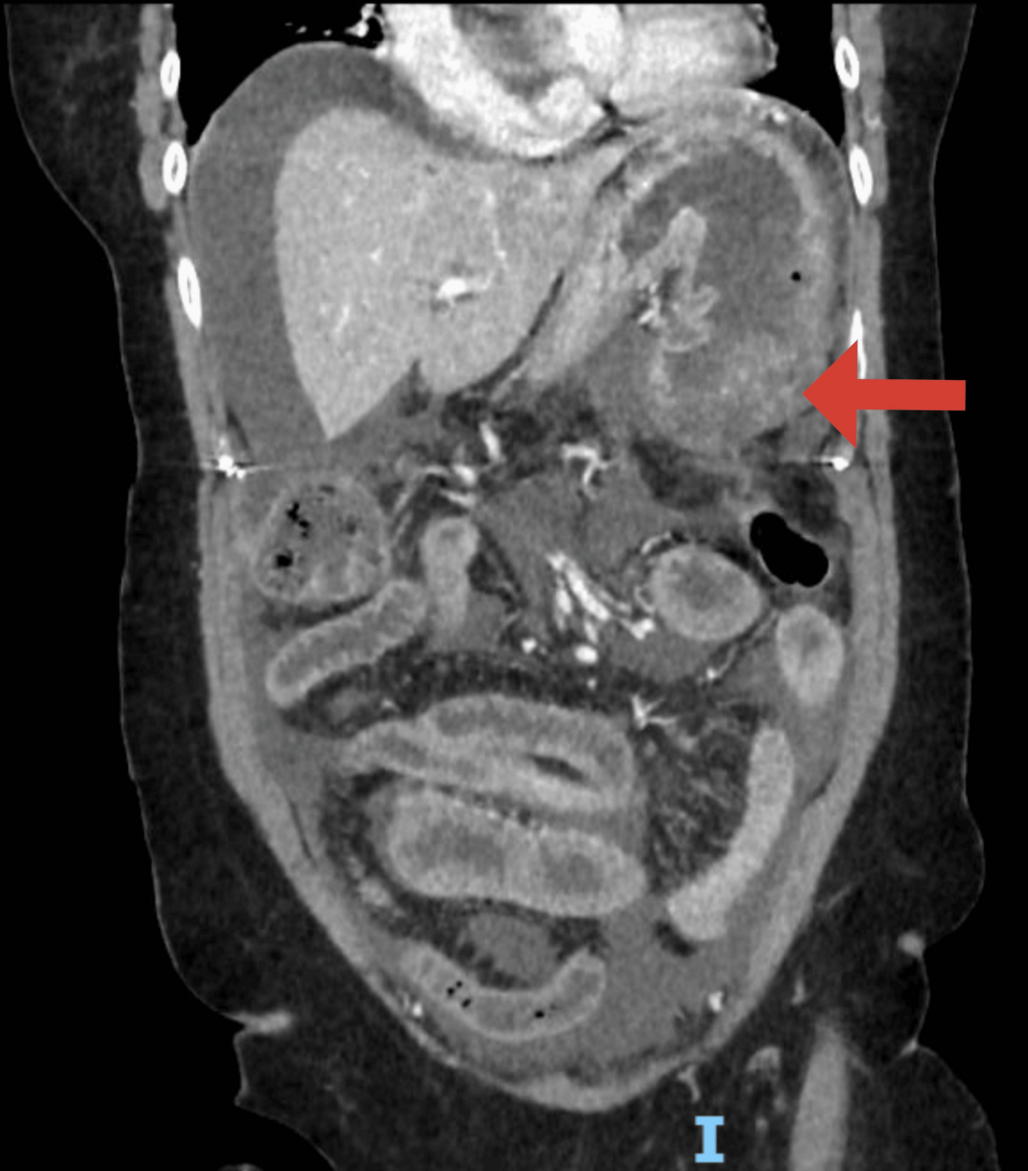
Contrast-enhanced abdominal computed tomography scan demonstrating the site of the gastric wall disruption (red arrow).
